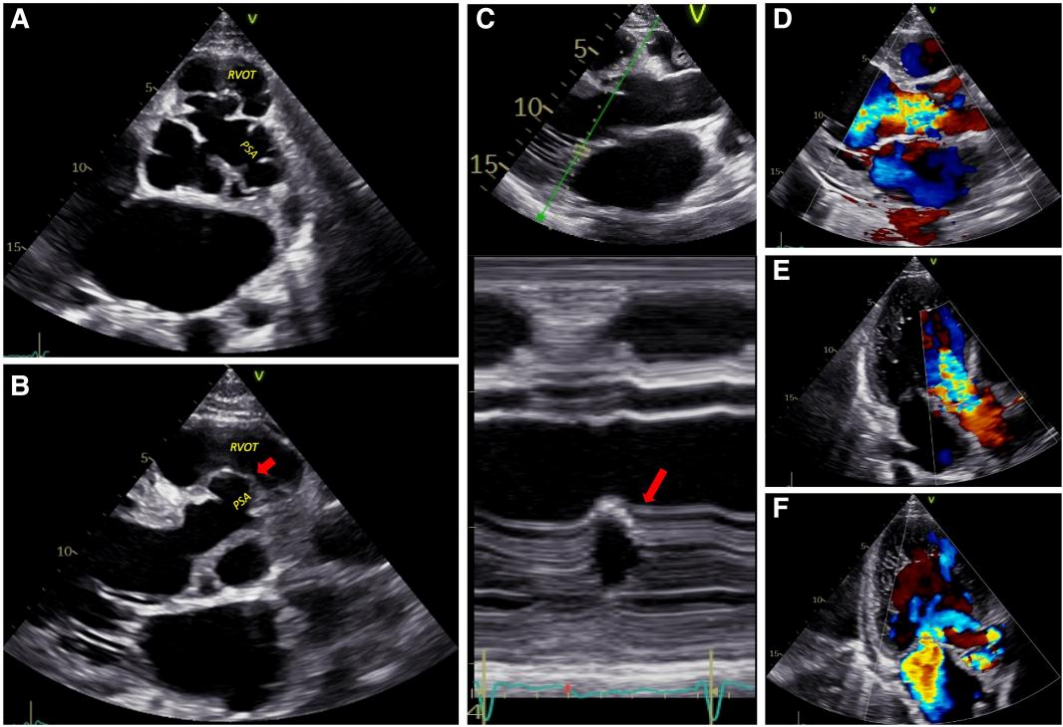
(*A*) Transthoracic parasternal short-axis view showing a multiloculated cavity consistent with a pseudoaneurysm of the ascending aorta. (*B*) Parasternal long-axis view revealing the pseudoaneurysm with a thin wall at the level of the right ventricular outflow tract. (*C*) M-mode echocardiography demonstrating early mitral valve closure, indicative of severe aortic regurgitation. (*D*) Parasternal long-axis view showing severe aortic regurgitation. (*E*) Apical three-chamber view showing severe aortic regurgitation. (*F*) Apical three-chamber view showing moderate mitral regurgitation. PSA, pseudoaneurysm; RVOT, the right ventricular outflow tract.
